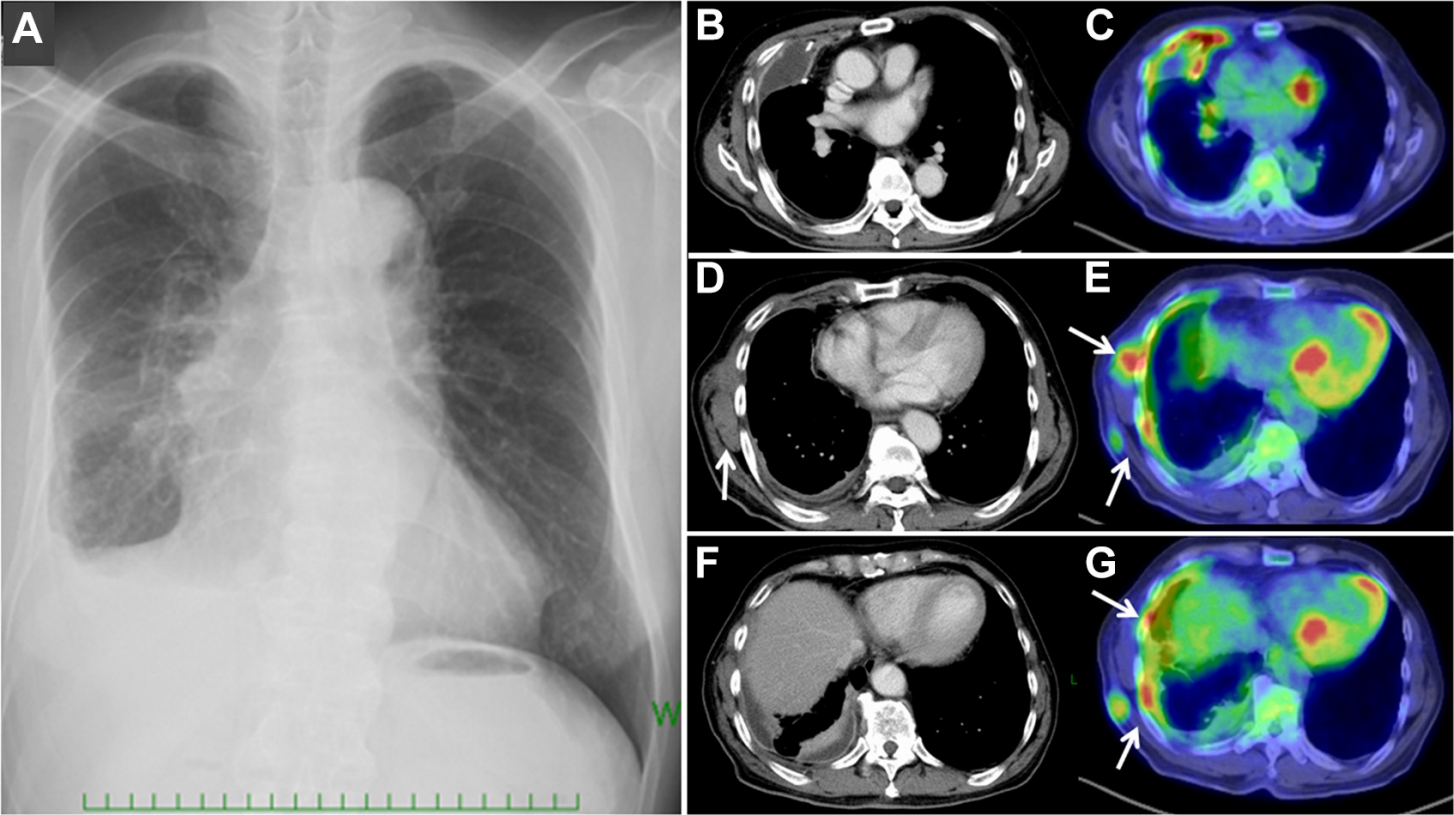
(A-G) Pretreatment imaging. (A) Chest roentogenogram, (B), (D), and (F), computed tomography, and (C), (E), and (G), positron emission tomography combined with computed tomography. Multifocal chest wall involvement was documented (arrows).
